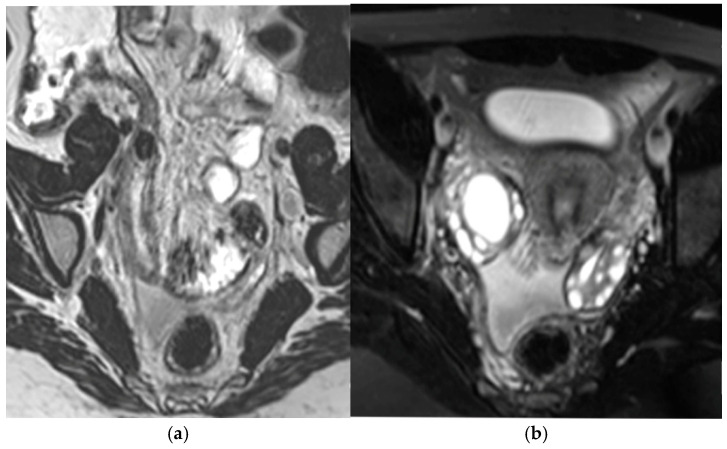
Acute appendicitis in a 15-year-old female presenting with acute pelvic pain. Previous US examination demonstrated a fluid right adnexal cyst, without other significant findings (a) Coronal T2-weighted HASTE image shows a tubular structure with thickened walls in the right iliac fossa, containing intraluminal coprolites. (b) Axial FS T2-weighted HASTE image confirms the inflamed appendix, with surrounding inflammatory changes and pelvic free fluid. MRI findings were consistent with acute appendicitis and were subsequently confirmed surgically.
